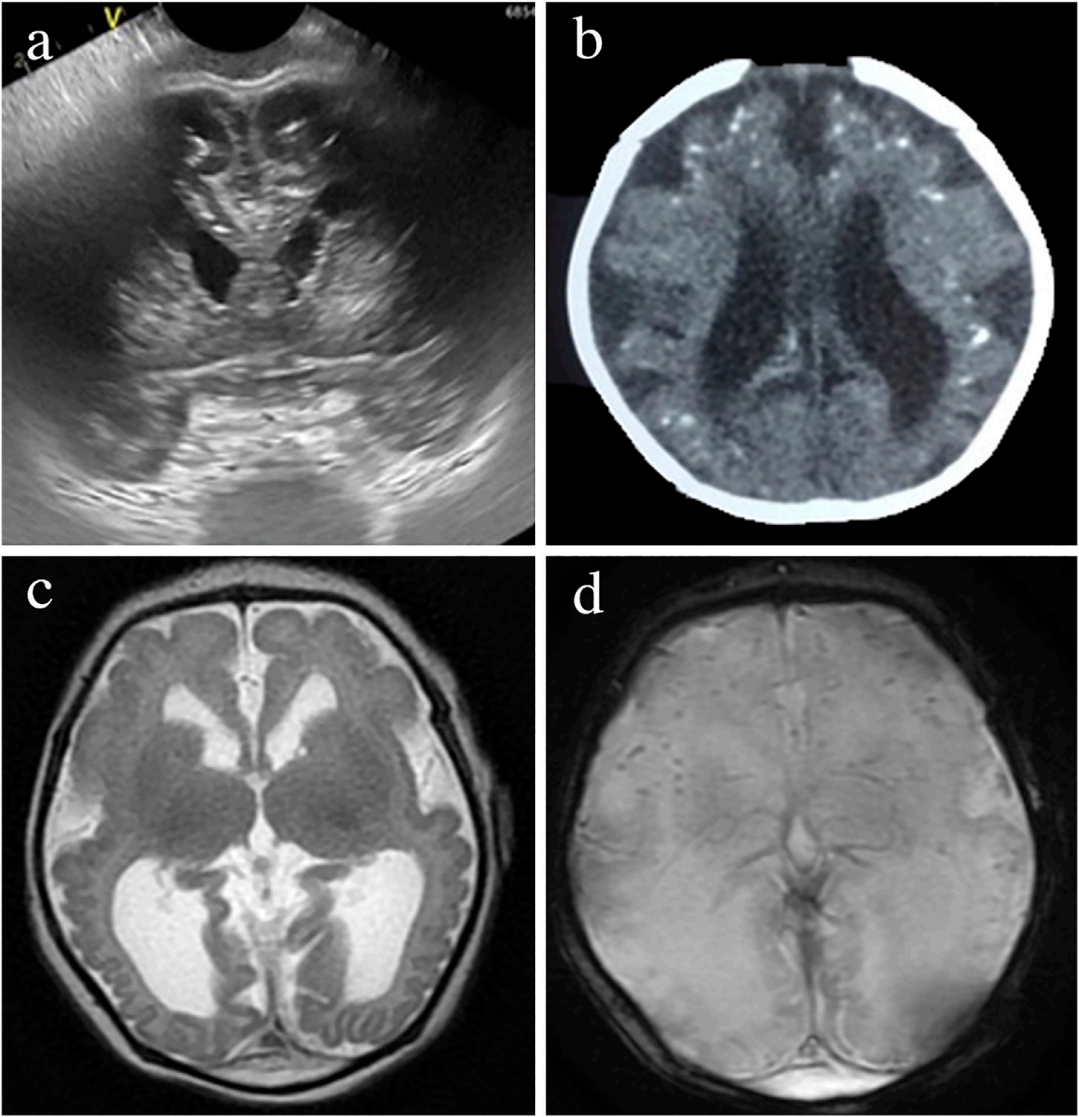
Brain neuroimaging studies in an infant with microcephaly and prenatal Zika infection. Head ultrasound image of a prefrontal coronal plane showing dilated frontal horns and cerebral calcifications below the subcortical border (A). CT axial image showing ventriculomegaly, calcifications predominantly located in the cortical-subcortical border and simplified gyral pattern (B). MRI- T2-weigthed axial image showing a very simplified frontal gyral pattern, ventriculomegaly, germinolytic pseudocysts in the caudothalamic groove, an open operculum, reduced white matter volume and absence of myelin in the posterior limb of the internal capsule (C). Gradient Echo T2* axial MRI image showing cerebral calcifications seeb as small, rounded, homogeneous, dark dot-like lesions (D).
